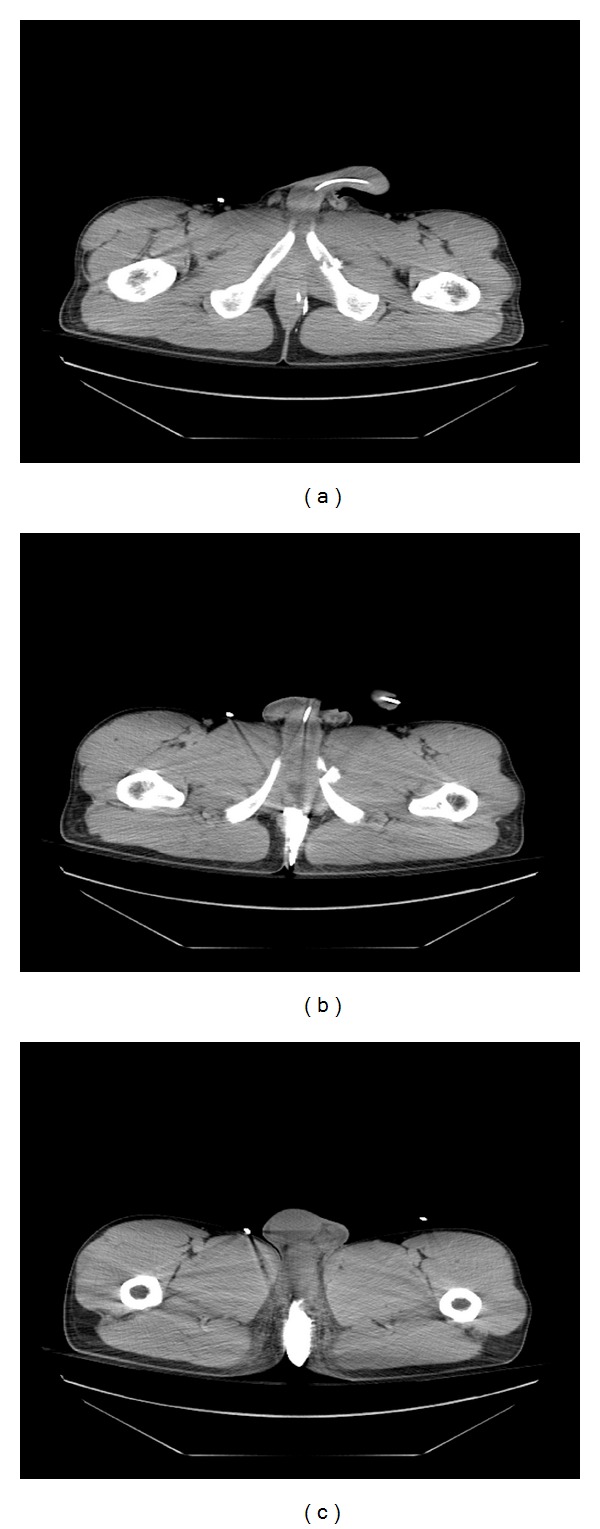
CT Urethrocystography (Siemiens). Contrast fills urethra without filling bladder. Left pubic bone has been detected in (a) and (b).
